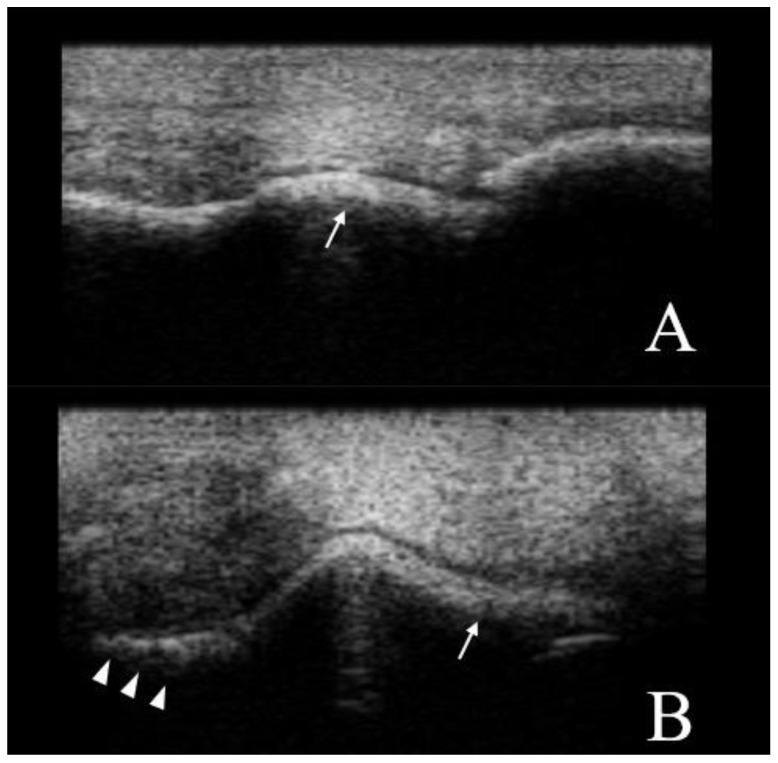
Ultrasound views of a standardbred MCP joint. (A) Dorsal longitudinal view, and (B) transversal view. The subchondral bone is irregular (arrow heads), showing a sclerotic appearance and shaded subchondral bone-cartilage interface (arrows). Proximal and lateral is to the left. B-mode, linear probe, 7.5 MHz.
